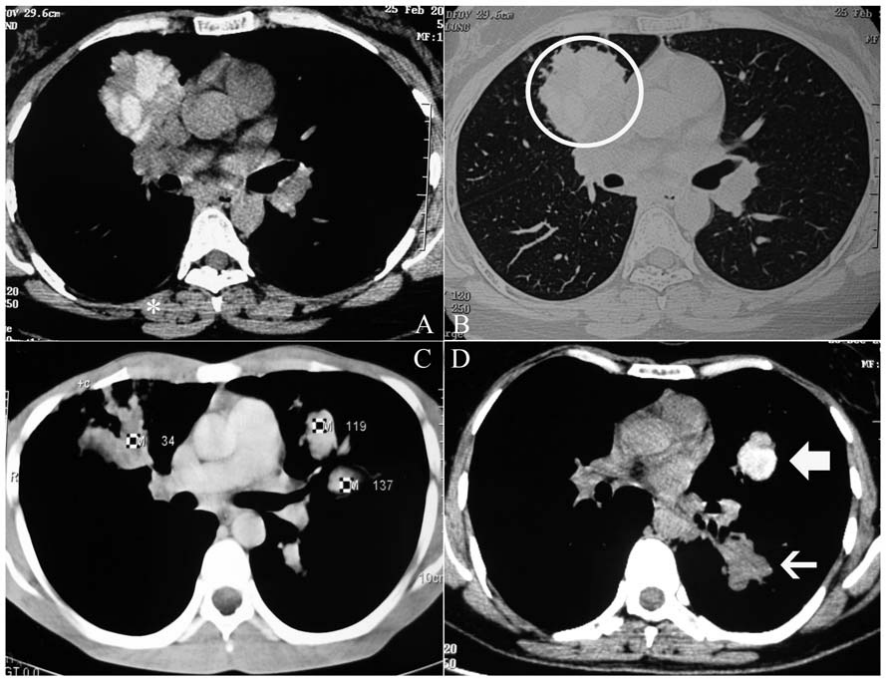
High-resolution computed tomographic images of patients with allergic bronchopulmonary aspergillosis demonstrating the presence of high-attenuation mucus. (A) Mediastinal window showing the presence of hyperattenuated mucus within dilated bronchi. The mucus is denser than the paraspinal skeletal muscle (asterisk) (B) Lung window shows that hyperdense mucus can occasionally be appreciated even with the parenchymal sections (circle); (C) CT Hounsfield values of mucus in dilated bronchi: mucus in the left lung is hyperdense with higher CT attenuation values compared to mucoid impaction in the right lung; (d) Hyperattenuated (bold arrow) and normal attenuation mucus (thin arrow) in the same mediastinal window.
